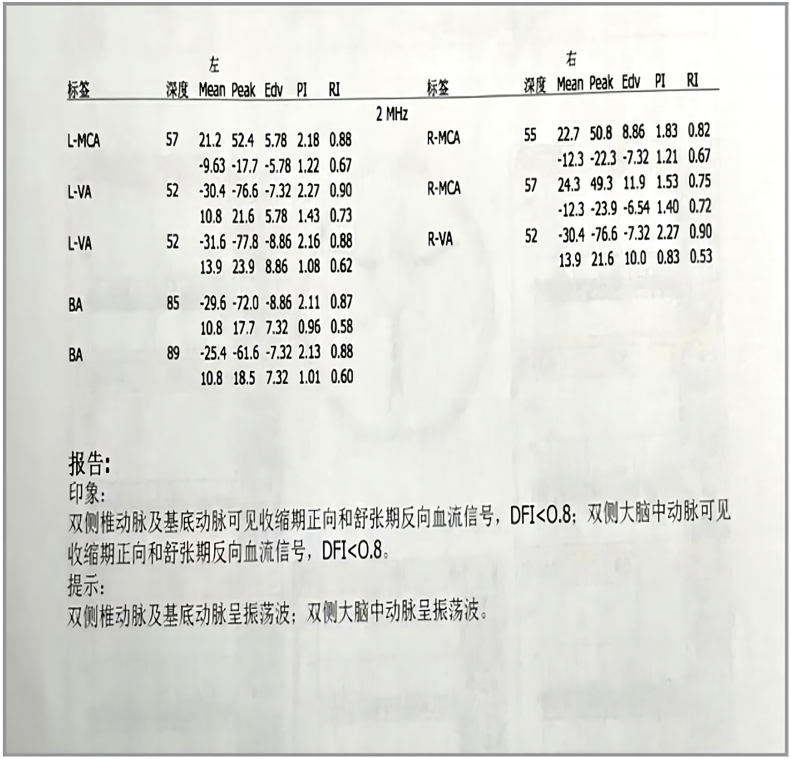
Transcranial Doppler (TCD) report.
